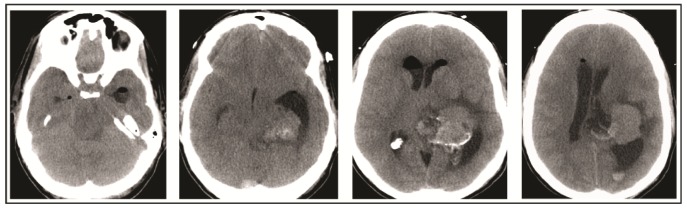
Head computed tomography (CT) performed on post-operative day 3 demonstrates cerebral edema and hydrocephalus.
